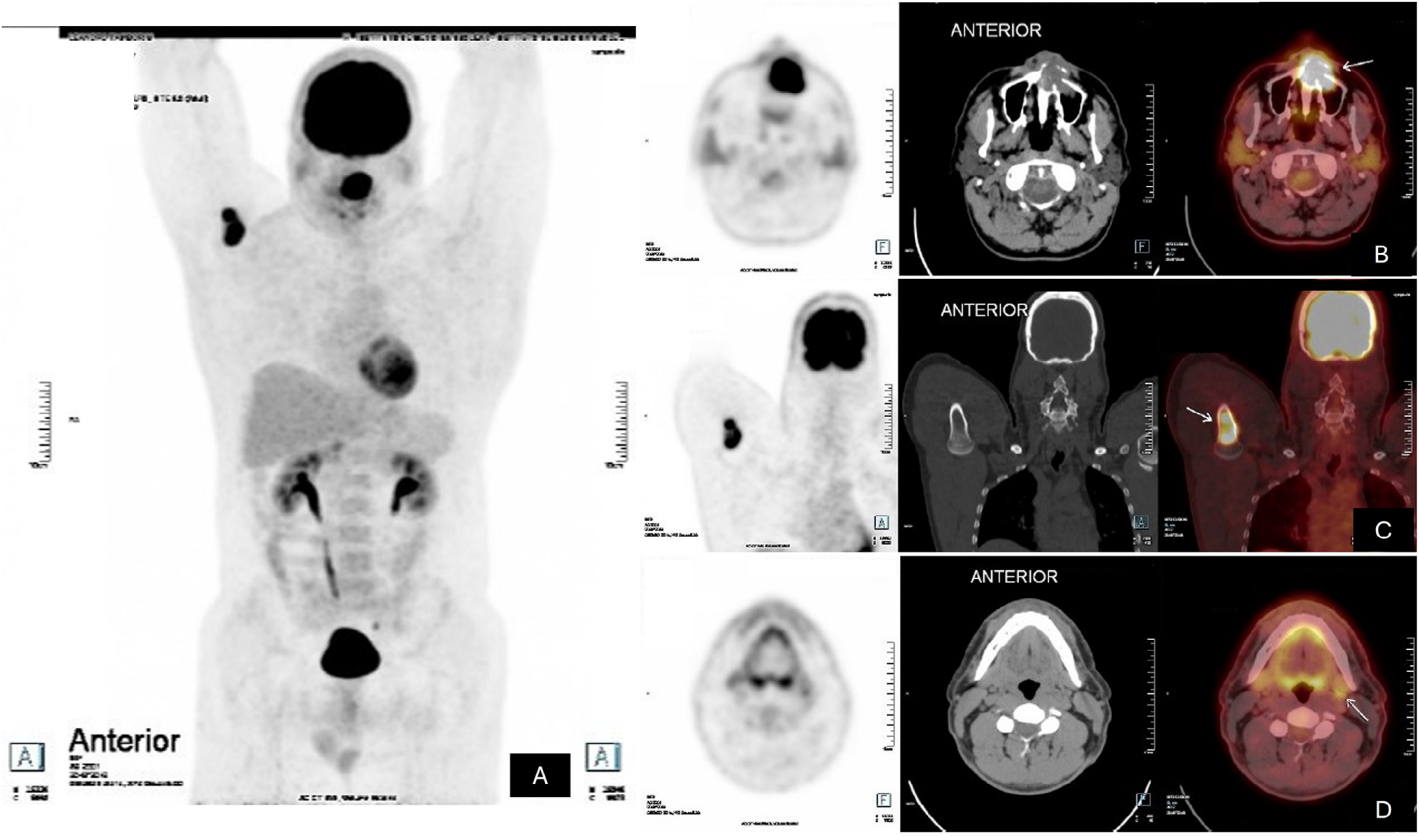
PET scan findings. (A) Whole‐body 18F‐FDG PET/CT scan revealing increased radiotracer uptake in the maxilla, right humerus, and left cervical lymph node. (B) PET/CT scan demonstrating a hypermetabolic lesion in the left maxilla, associated with bone destruction and invasion of the inferior portion of the nasal cavity and the ipsilateral maxillary sinus. (C) Hypermetabolic medullary lesion in the proximal third of the right humerus. (D) Hypermetabolic lesion in the left cervical lymph node.
